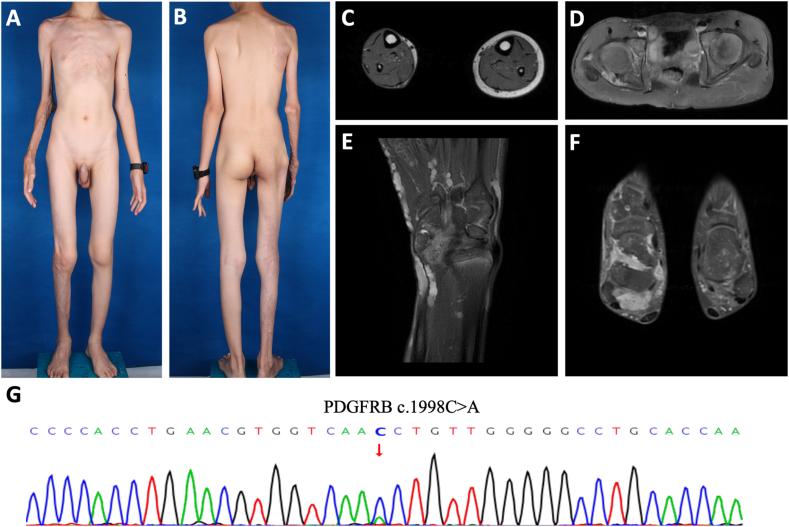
The patient's phenotypic and genetic features. (A, B) Anterior and posterior views of the patient showing right-sided hemilateral adipose and muscular atrophy, with prominent superficial veins and an increased arm span-to-height ratio. (C) Axial T1-weighted MRI showing reduced subcutaneous fat and muscle bulk in the right limbs. (D–F) MRI revealed multiple vascular lesions in the right limbs, presenting as heterogeneous enhancement on contrast-enhanced T1-weighted images (D, F) and high signal intensity on T2 fat-suppressed imaging (E). (G) Validation of the *PDGFRB* c.1998C>A (p.N666K) variant by Sanger sequencing.
